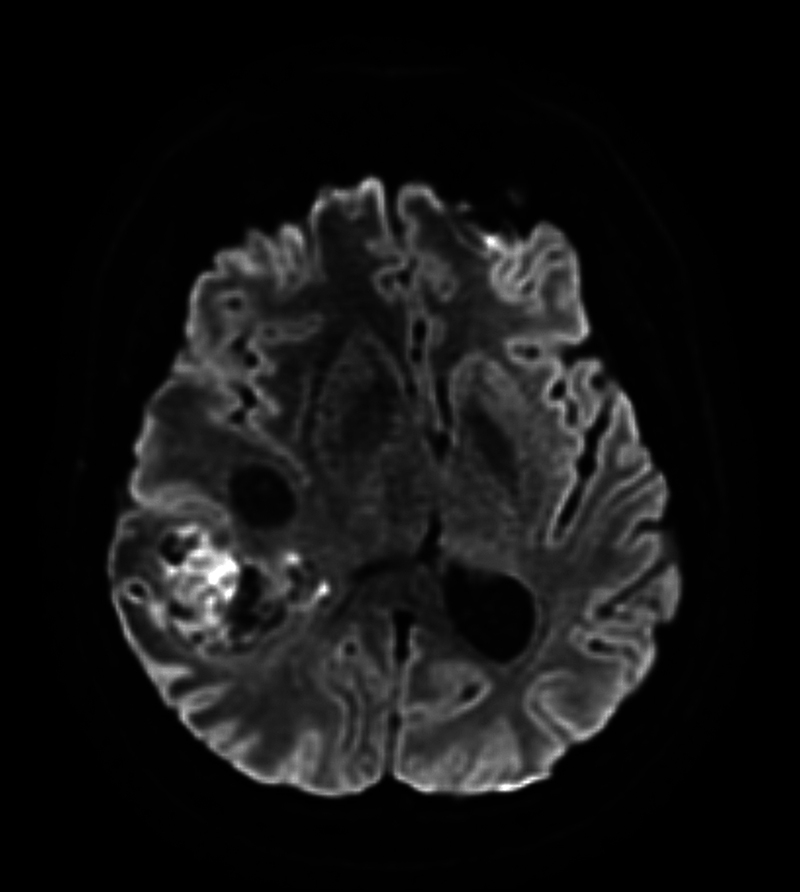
Axial T2-weighted diffusion-weighted imaging (DWI) of the glioblastoma shown in Figs. 1 and 2. The hyperintense signal within the tumor indicates areas of hypercellularity and restricted water diffusion.
